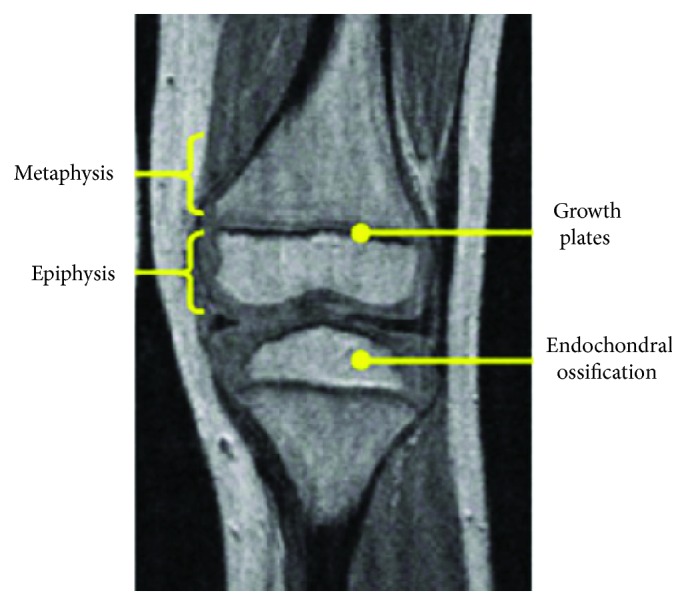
Child long bones MRI (figure based on [24]).
